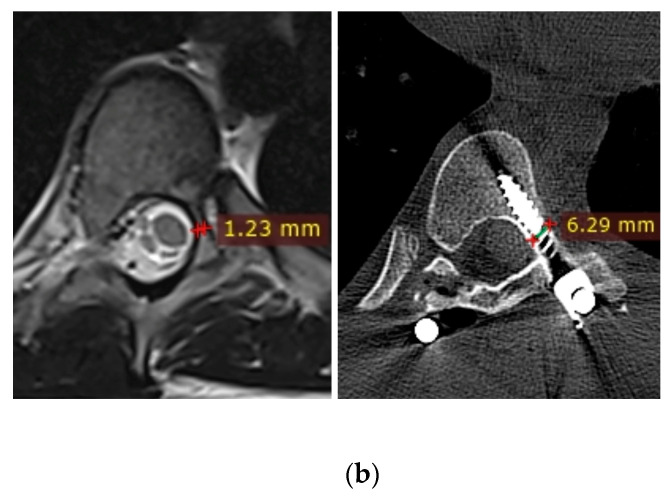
(a). Measurement of vertebral pedicle transverse diameter (A,B) in a magnetic resonance axial cut image. (b). Increasing screw size in small pedicles, showing the widening of vertebral pedicle transverse diameter without bone fracture. Preoperative images were screened via MRI and compared to the same levels with screws inserted using postoperative CT screening.
